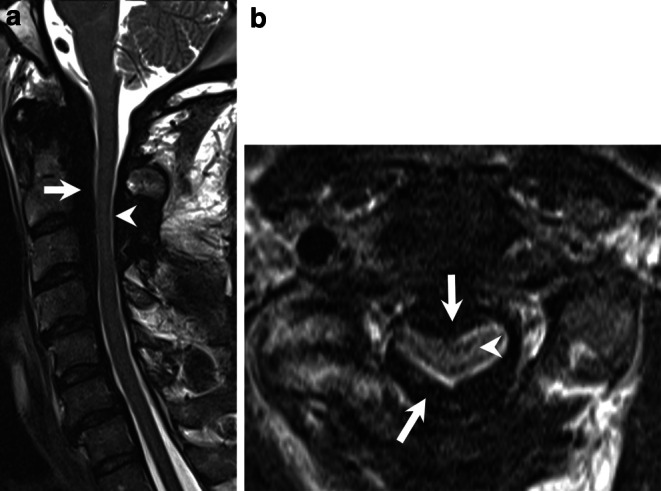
Sagittal (a) and axial (b, level C2/3) T2-weighted images showed a high-grade stenosis of the cervical canal between C2 and C4 (a, *arrowhead*). The stenosis was caused by a marked enlargement of the dura/epidural space extending from the foramen magnum down to C7. On T2-weighted images, the space-occupying lesion was hypointense (a, b, *arrows*). In addition, a centromedullary hypersignal corresponding to a cervical myelopathy was present (b, *arrowhead*)
